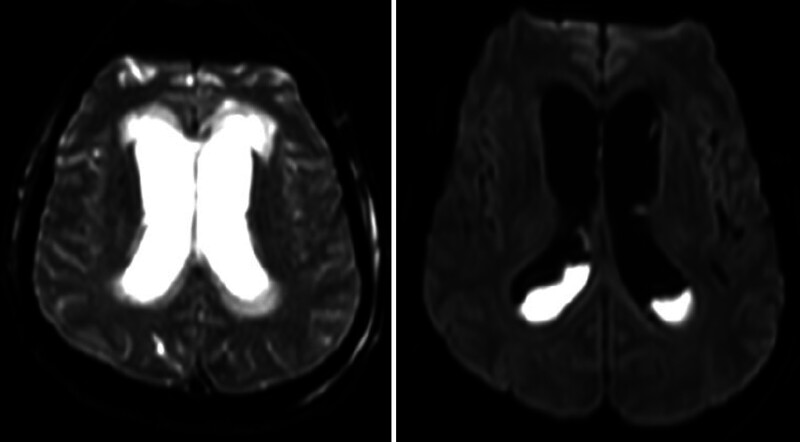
MRI brain confirmed progressive hydrocephalus with intraventricular hemorrhage.
